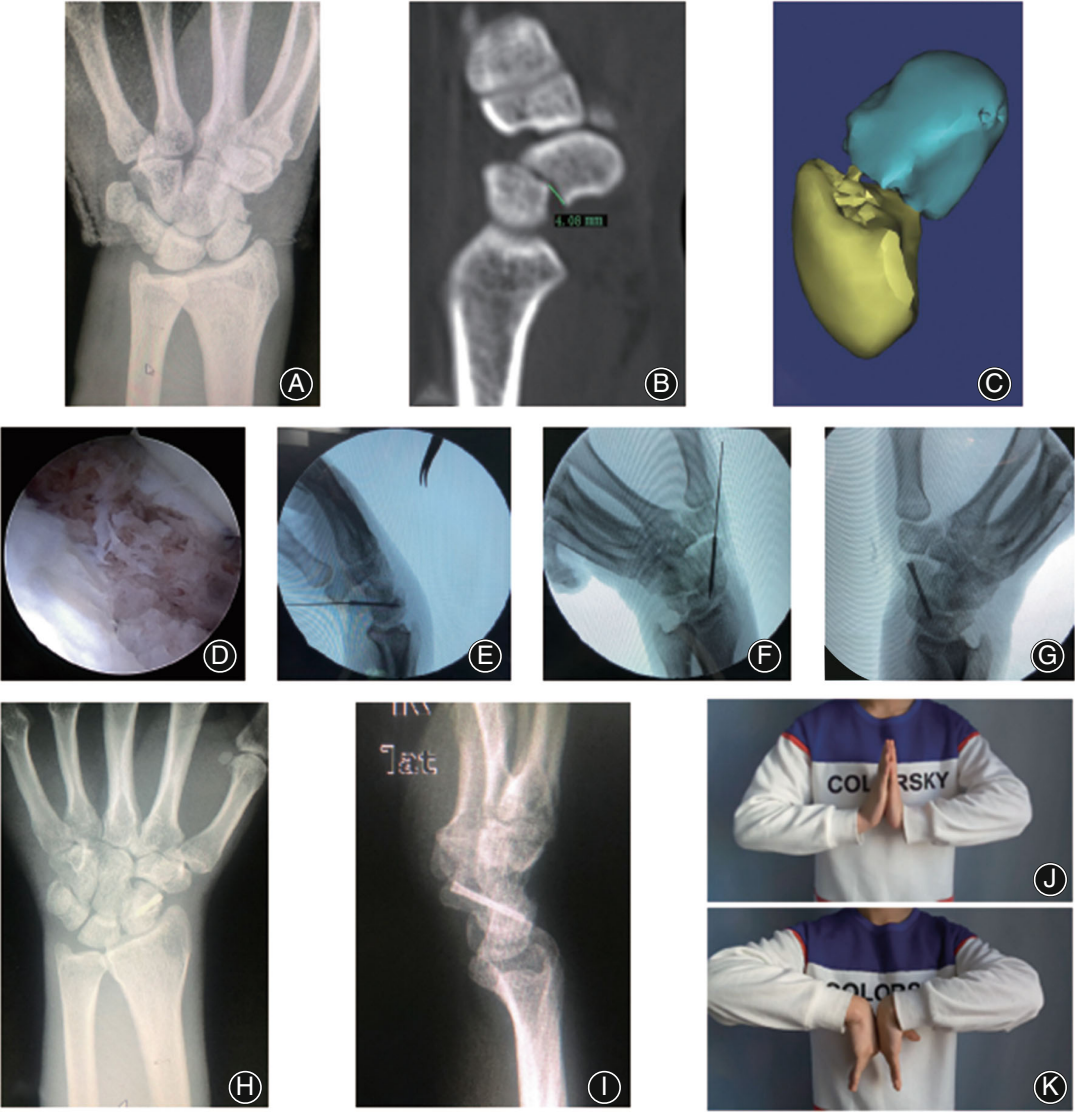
Medical imaging for case 2: A 29‐year‐old male with pain, swelling, and limited activity of the right wrist for 1 day caused by falling from a height. (A) Preoperative CT indicated right carpal scaphoid Herbert B2‐type fracture; (B, C) Preoperative CT and three‐dimensional reconstruction showed that the displacement of carpal scaphoid fracture was obvious, and the fracture step reached 4.08 mm; (D) fracture reduction with arthroscopically assisted procedure; (E, F, G) The screw was inserted along the guide wire, and the fracture and internal fixation were in good position under C‐arm fluoroscopy; (H, I) X‐ray image showed that the fracture line disappeared 15 months after the operation; (J, K) The patient had good wrist extension and flexion at 15 months postoperatively
